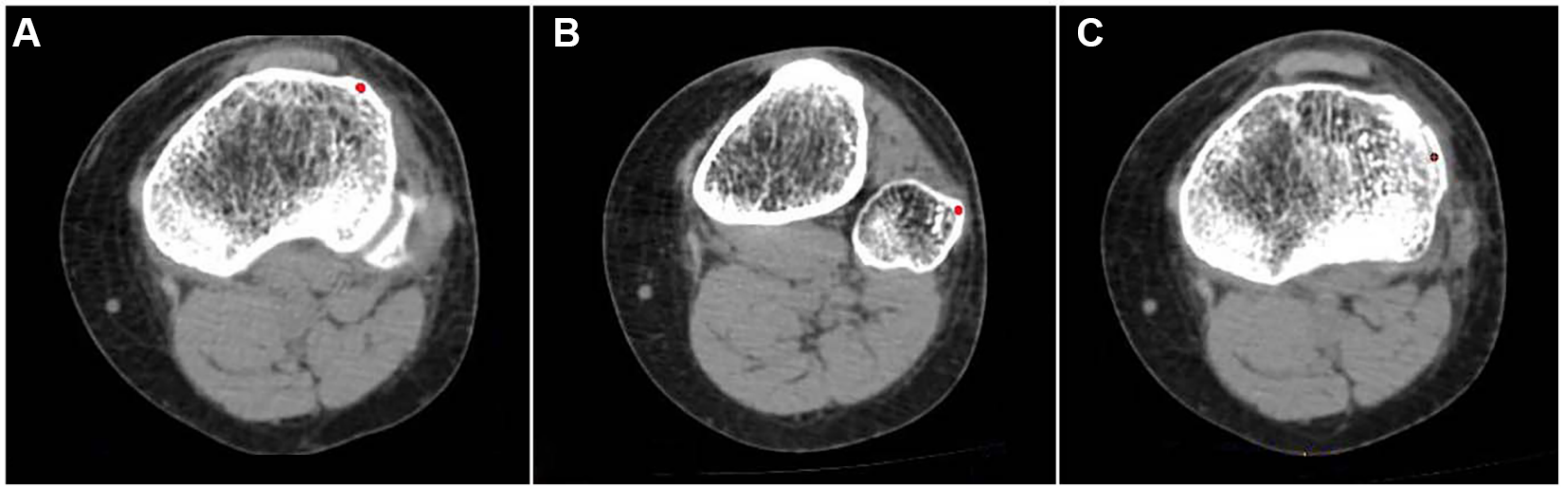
(A) Highest point of the Gerdy tubercle in the CT axial slices. (B) Outermost point of the fibular head in the CT axial slices. (C) Center of the Segond fracture bed in the CT axial slices. Red dots indicate reference points.
